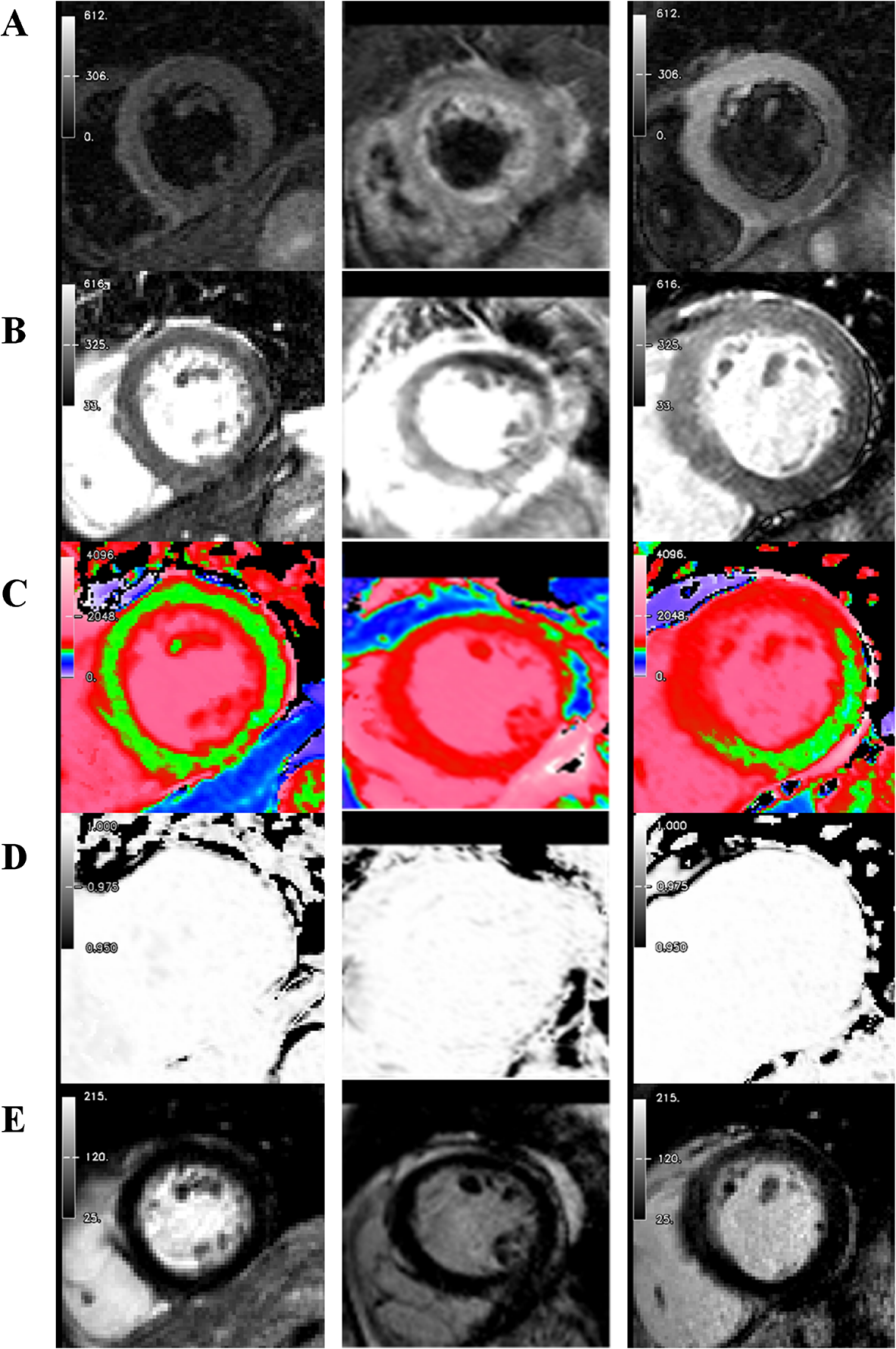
CMR imaging modalities used for comparison – representative images in short-axis slices from: (*Left panel*) Normal volunteer (*Middle panel*) Patient with Takotsubo cardiomyopathy. Note high T2 signal and T1 values in an apical slice. (*Right panel*) Patient with regional edema in the anterior wall territory. Note high T2 signal and T1 values in the anterior, anterolateral wall and anterior septum. Rows: (A) Dark-blood T2-weighted images (reference ROIs in skeletal muscle and remote myocardium not shown). (B) Bright-blood T2-weighted images. (C) Colour ShMOLLI T1-maps. Green denotes normal myocardium. Red denotes increased T1 values (D) R^2^ maps of ShMOLLI inversion recovery fit used to verify the quality of T1-maps. (E) LGE imaging.
